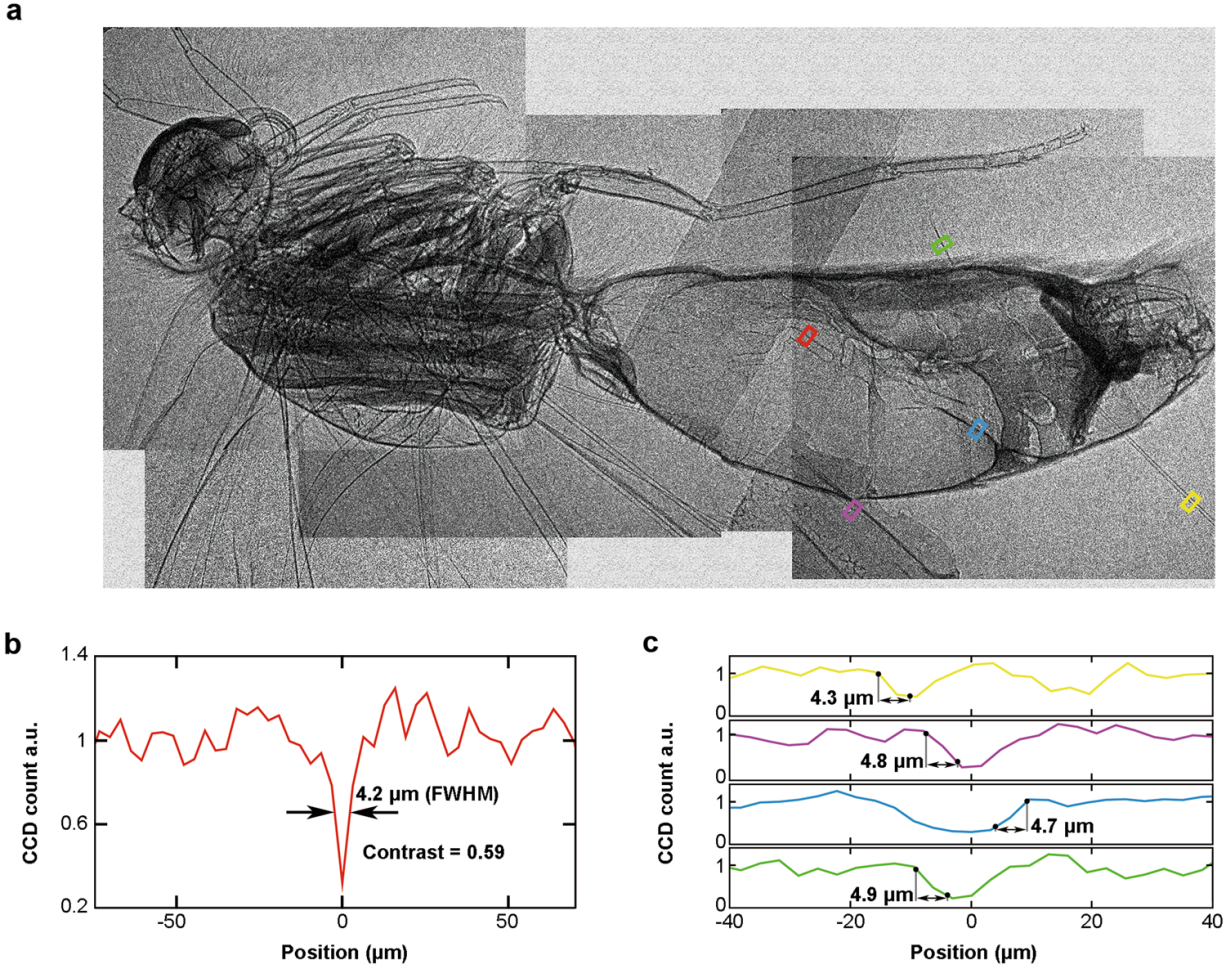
(a) X-ray phase contrast image of a 1-cm long butterfly under ionization injection condition, which is composed of 5 sub-images taken separately, and each sub-image was obtained by 10 exposures. (b) Lineout of the red square region in (a). A narrow dip of the gastrointestinal wall with a 4.2-*µ*m width (FWHM) can be clearly identified and measured with a contrast of 0.59. (c) Lineouts of the yellow, magenta, blue and green square regions in (a). The sharp edges with widths of ∼5 *µ*m (10% to 90% *H* − *L*) were measured.
